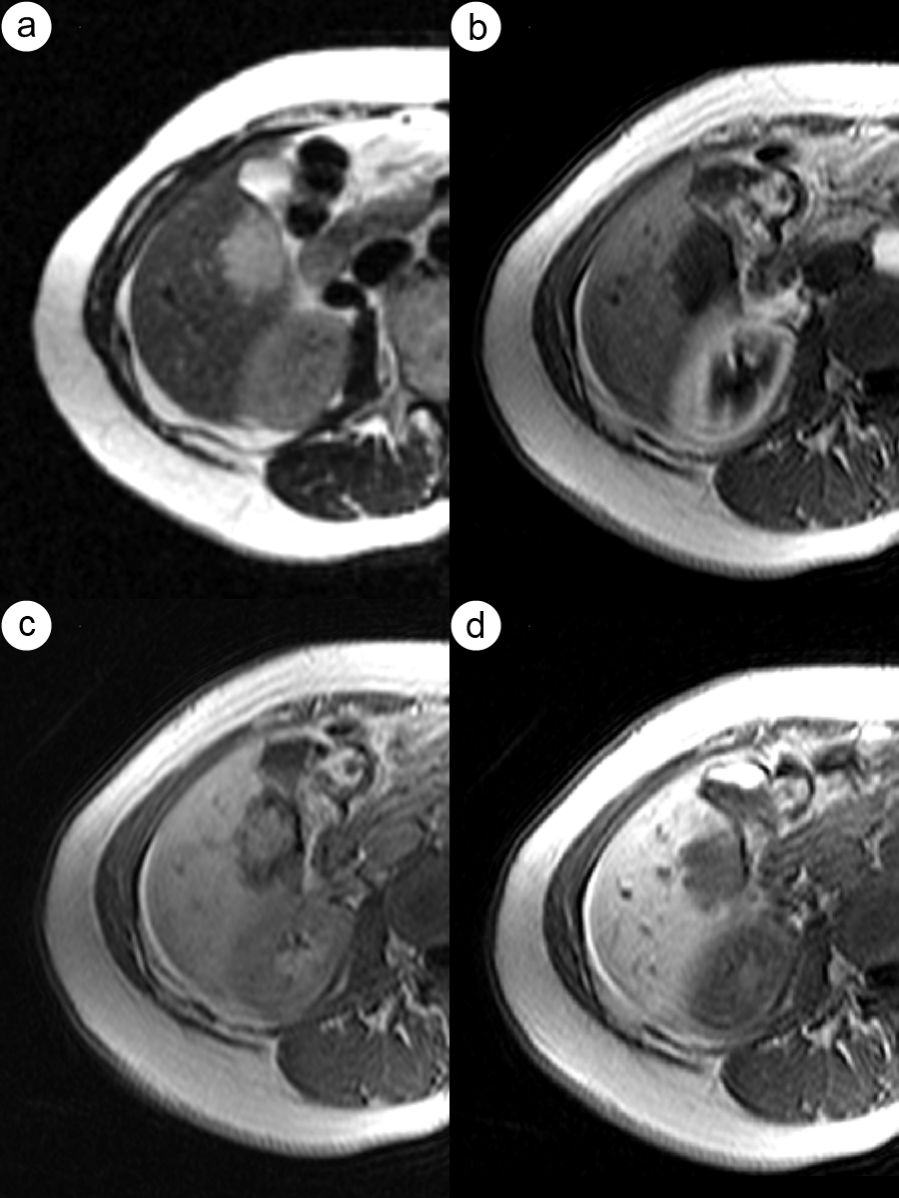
Hepatic hemangioma with atypical enhancement pattern in dynamic MR study. Moderately T2-weighted MR image with untypical weak intensity of the lesion (a). In HAP (b) enhancement of the hemangioma is not visible. Figure 6c shows central enhancement of the lesion in EP. The hemangioma presents weaker enhancement than the adjacent liver parenchyma in hepatobiliary phase (d).
